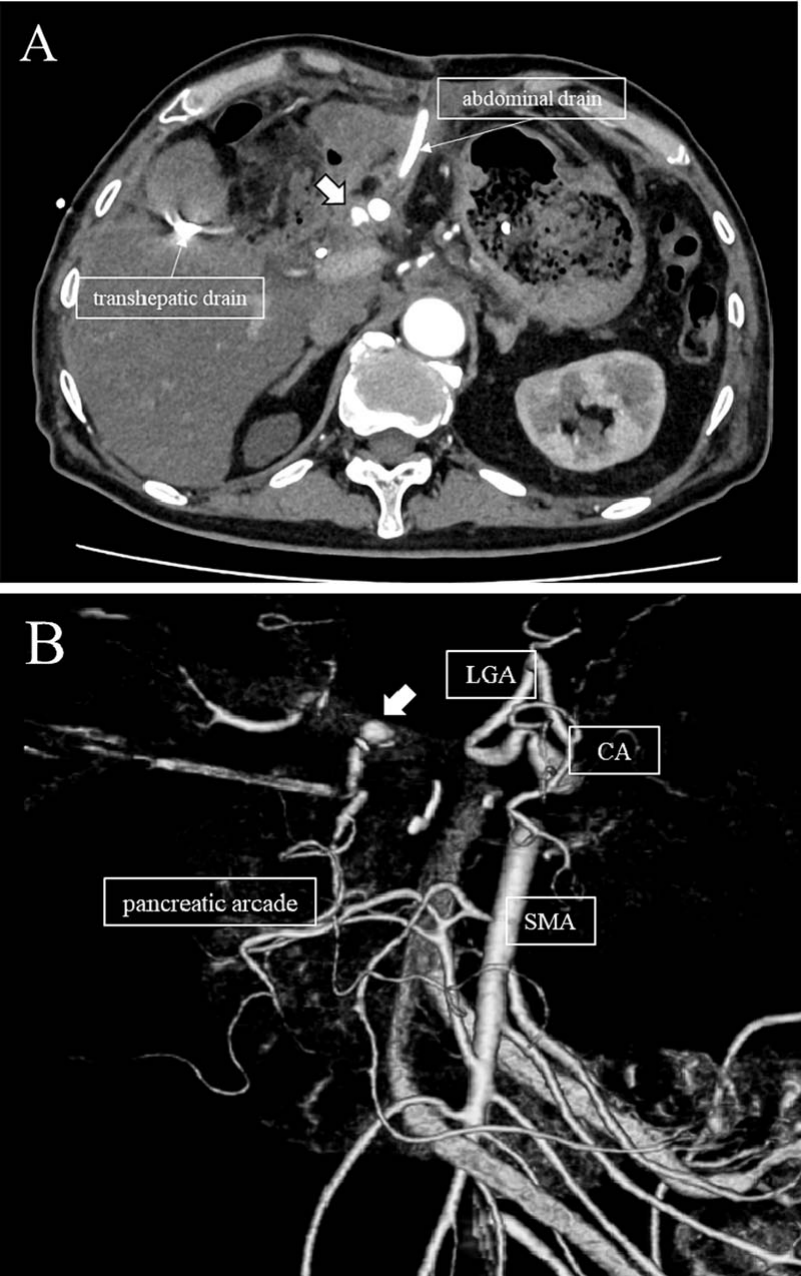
(A, B) CT on postoperative day 48. Contrast-enhanced CT showing a pseudoaneurysm at junction of the GDA and PHA (arrow).
